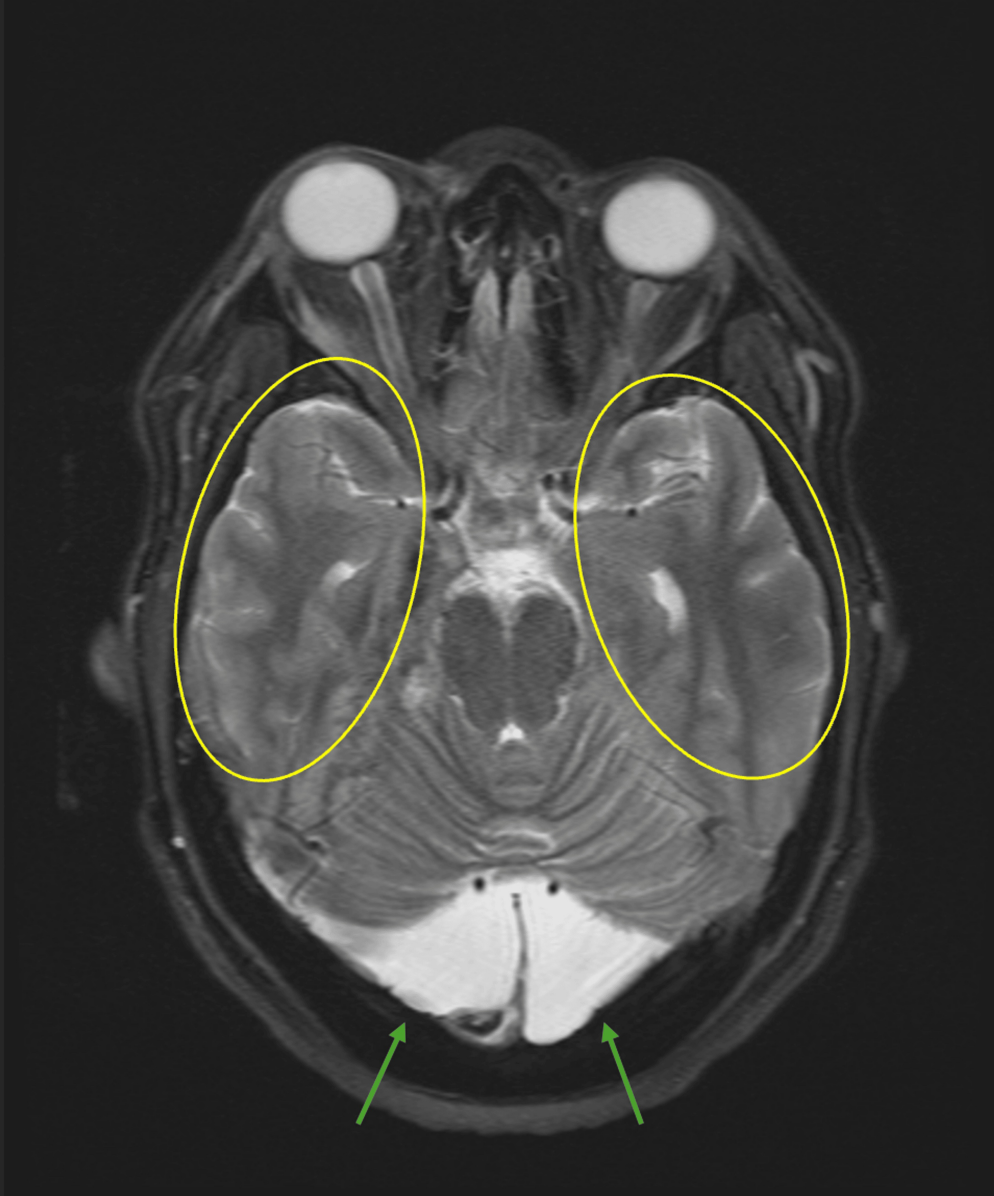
MRI brain: T2-weighted. The yellow circles highlight extensive leptomeningeal enhancements involving the anterior aspects of the posterior fossa. The green arrows point to a CSF intensity area in the posterior cranial fossa measuring 3.7 x 5.9 x 4.9 cm, which is favored to represent a mega-cisterna magna.
